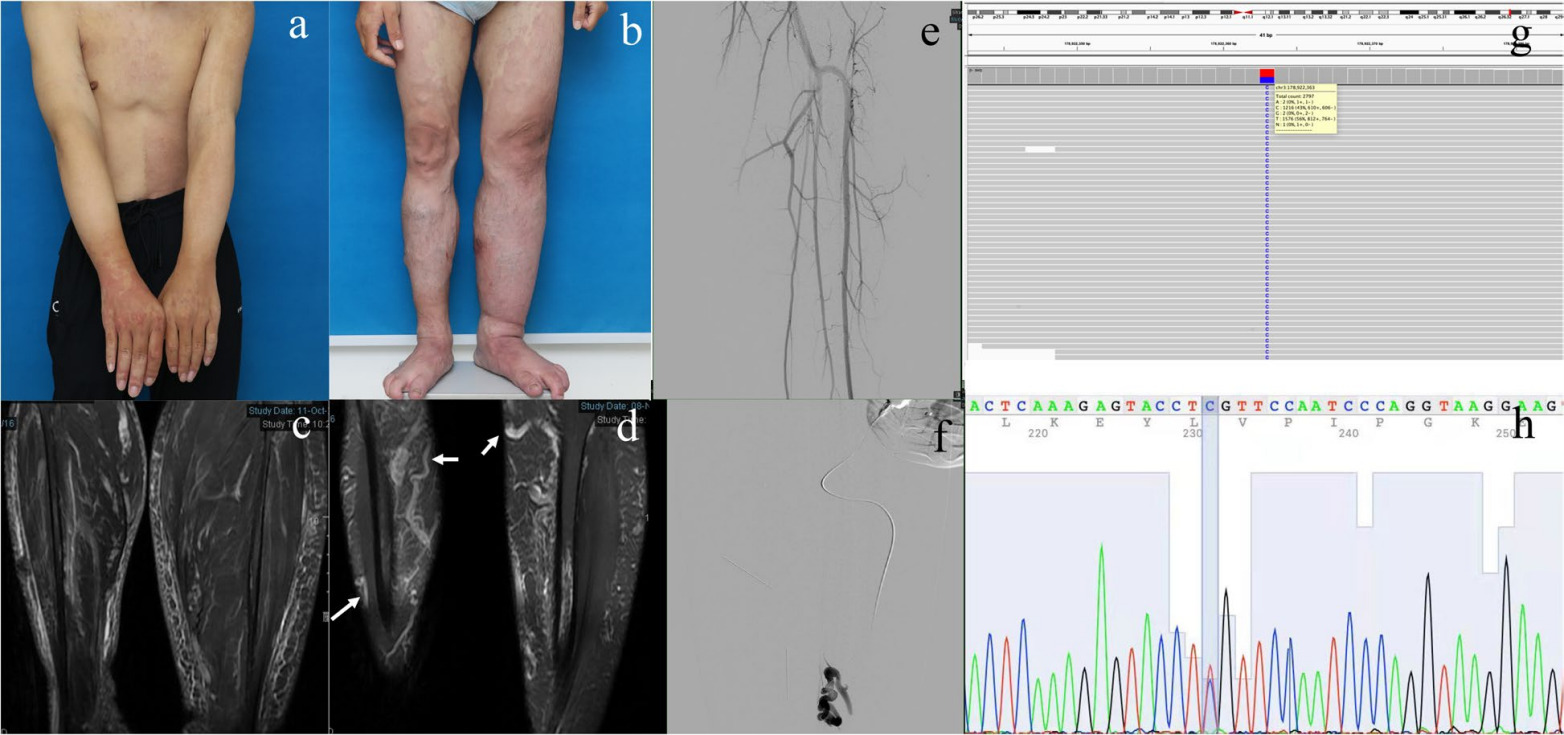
Clinical manifestation of the patient. a: Extensive, bilaterally and segmentally distributed capillary malformation on both the lower and upper limbs, chest and back. b: Bilateral hypertrophy associated with longitudinal growth, peripheral venous dilatation and increased skin temperature of the lower extremities. c-d: MR imaging showed diffuse tissue hypertrophy (1c) and multiple venous dilation (1d, arrows) of soft tissue and muscle of bilateral lower limbs. e: Intraarterial digital subtraction angiography (IADSA) showed no obvious AVM/AVF. f: Intravenous DSA (IVDSA) showed venous dilation of the soft tissue and muscle. g: IGV visualization of NGS data for the tissue sample with somatic mutation in *PIK3CA* (p.Cys378Arg; c.1132 T > C). h: Sanger sequence of somatic mutation in *PIK3CA* (p.Cys378Arg; c.1132 T > C)
